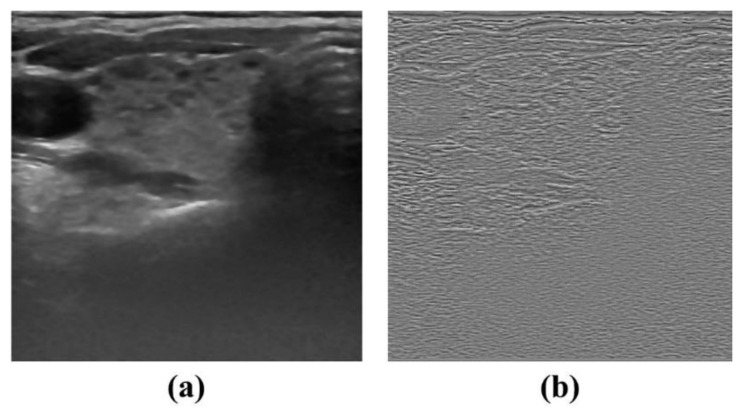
NLM denoising results using default standard deviation value (*δ* = 20) for (a) denoised ultrasound image and (b) residual image.
